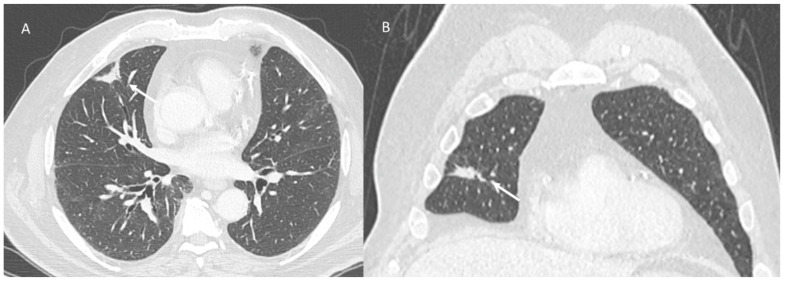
Radiation recall pneumonitis (CT scan axial: (A) and coronal: (B)) pattern includes consolidative opacities limited (arrows) to a prior radiation field.
